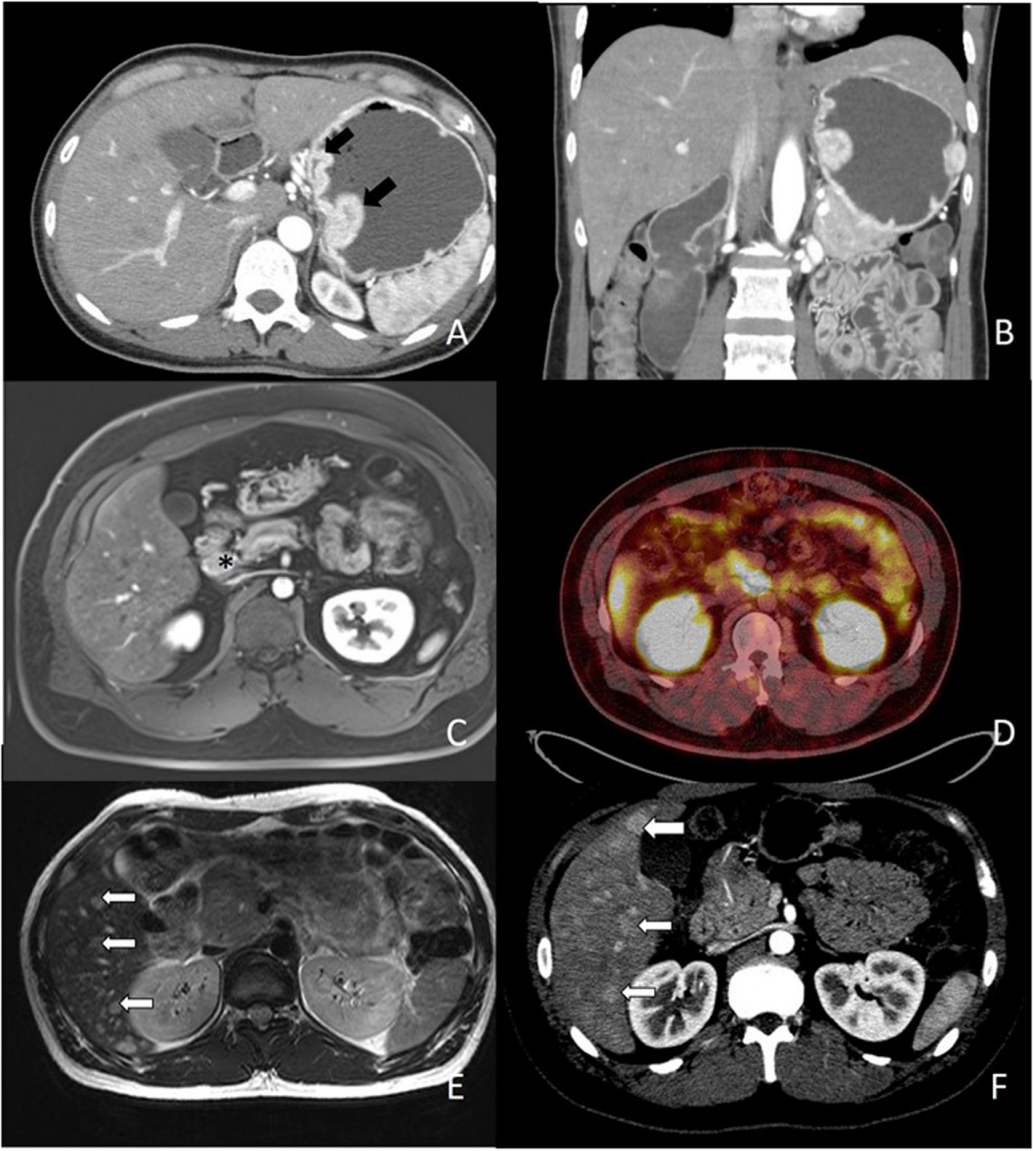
Axial contrast enhanced CT scan of upper abdomen (arterial phase) shows a two enhanced polypoid mass (*black arrow*) at high gastric body along lesser curvature side. b Coronal multiplanar reformation image. (1) Axial dynamic fat-saturated T1-weighted image with gadolinium enhancement shows enlarged lymph node (*) at peripancreatic area (c). (2) Ga-68 DOTATOC-scan shows strong uptake at medial aspect of uncinate process of pancreas (d). (3) Infiltrative tumor at pancreatic head with diffuse liver metastases (*white arrow*) e Axial dynamic fat-saturated T2-weighted image with gadolinium enhancement. f Axial contrast CT scan in arterial phase
